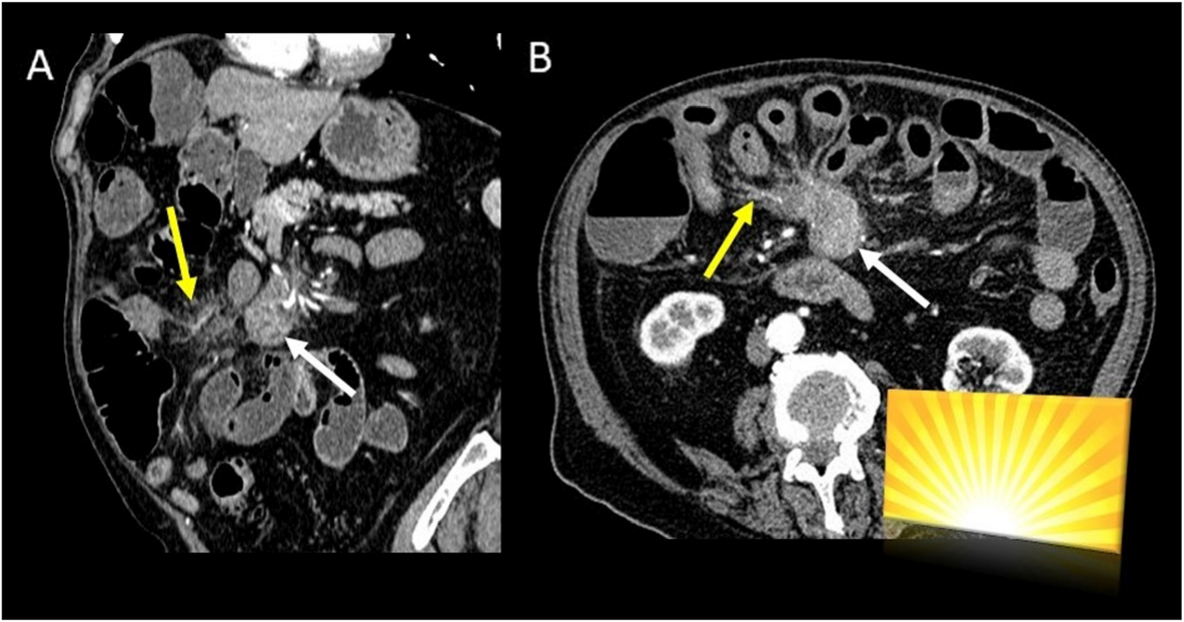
Mesenteric carcinoid tumor. CT images ((a) coronal and (b) axial plane) show hypervascular mesenteric mass (white arrow) with intralesional calcification and an intense fibrotic proliferation (yellow arrow), the so-called spoke-wheel or sunburst sign
